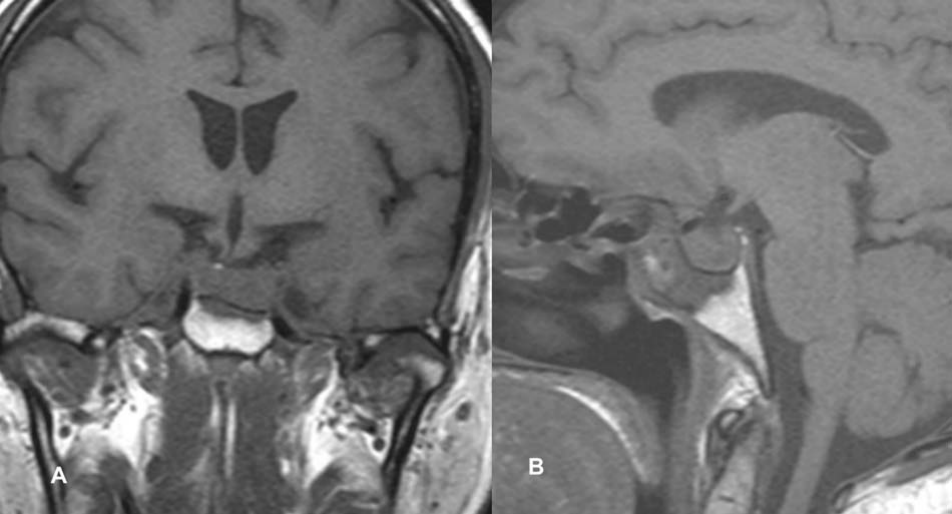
Follow-up MRI imaging of case 1 showed the sellar, suprasellar residual lesion measuring 1.9 × 1.5 × 1.1 cm without a significant mass effect on cavernous sinus.
